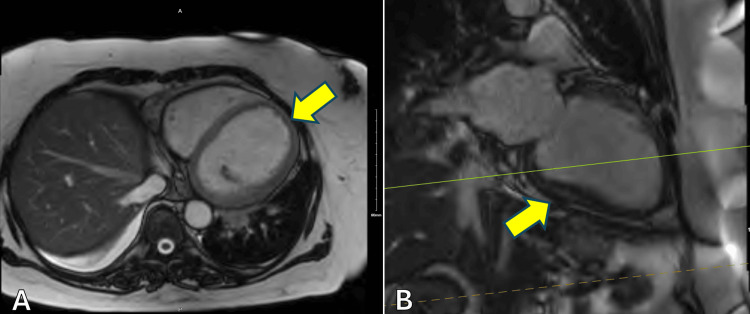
Cardiac MRI showing a dilated left ventricle with no left ventricular thrombus in axial (A) and sagittal (B) views. Left ventricle indicated by the yellow arrow. MRI, magnetic resonance imaging
